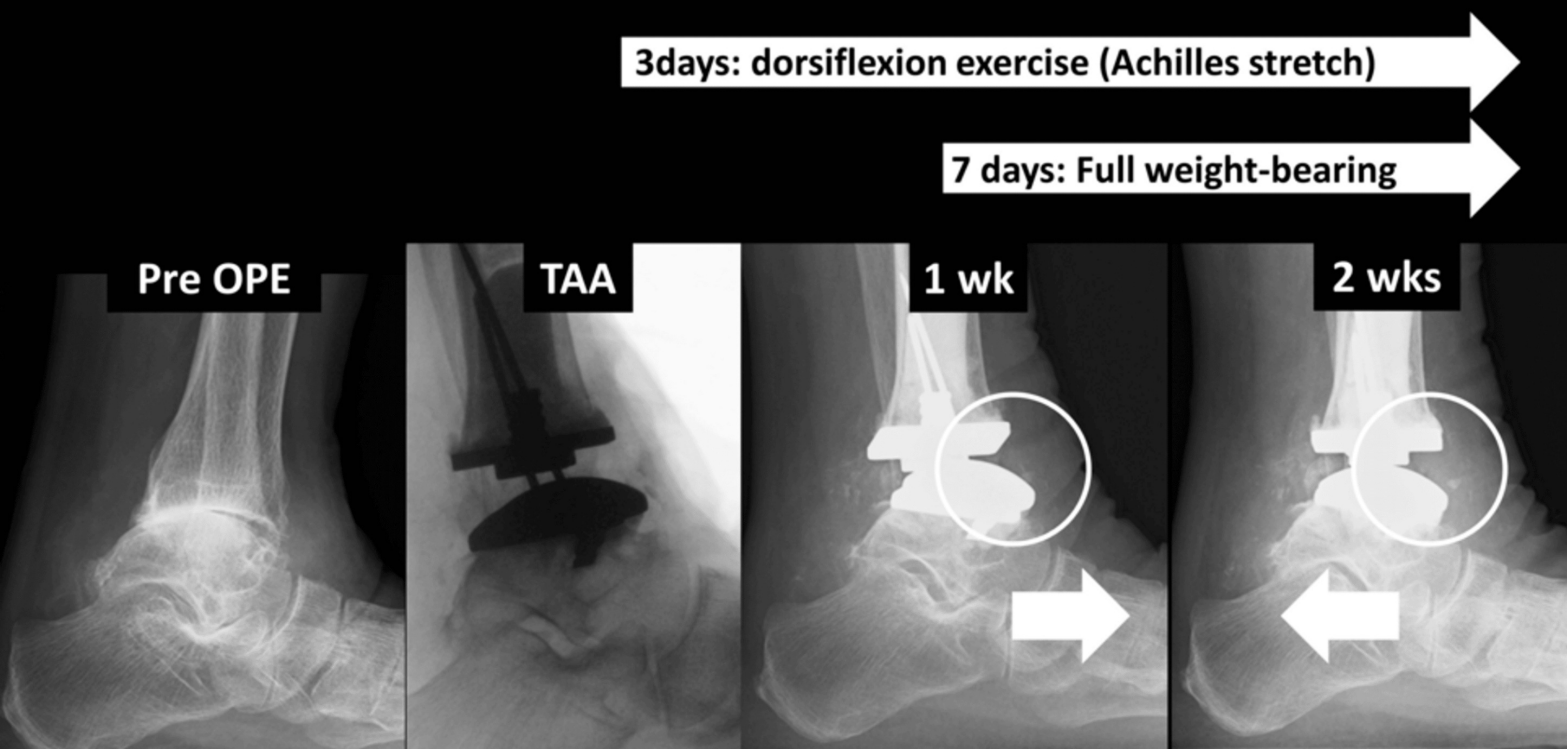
Transition of talar bone location during the perioperartive period in lateral view radiographs at weight-bearing Radiograph images belong to one of the patients in the current study. After TAA, the anterior shift of the talar bone remained for one week after surgery. However, Achilles tendon stretch by passive/active dorsiflexion three days after surgery and full weight-bearing/walking seven days after surgery contributed to relocation to the posterior side of the talar bone. This effect could contribute to the natural movement of the ankle joint.
